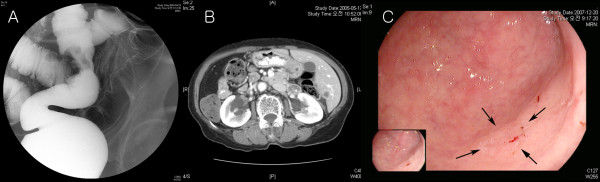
Metachronous double primary cancer in 71-year-old female patient with situs inversus totalis. a Barium double contrast study showing an ulcerative tumor in the rectosigmoid colon. b An abdominal computed tomography scan showing a single 9-mm metastatic nodule in the liver (segment 6) 14 months after laparoscopy-assisted low anterior resection. c Upper gastrointestinal endoscopy shows an ill-defined 5-mm mucosal lesion on the antrum 46 months after laparoscopy-assisted low anterior resection (black arrows).
